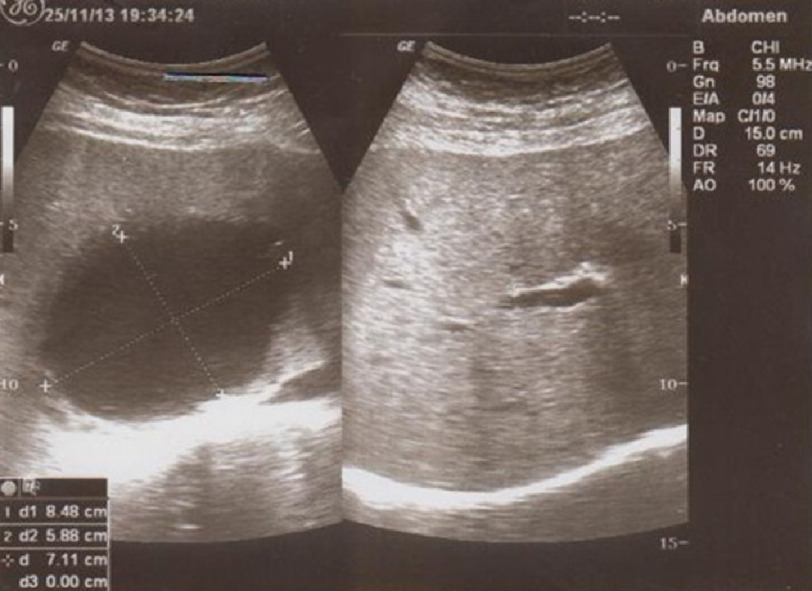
Abdominal sonography revealed a large cystic lesion in the liver.
